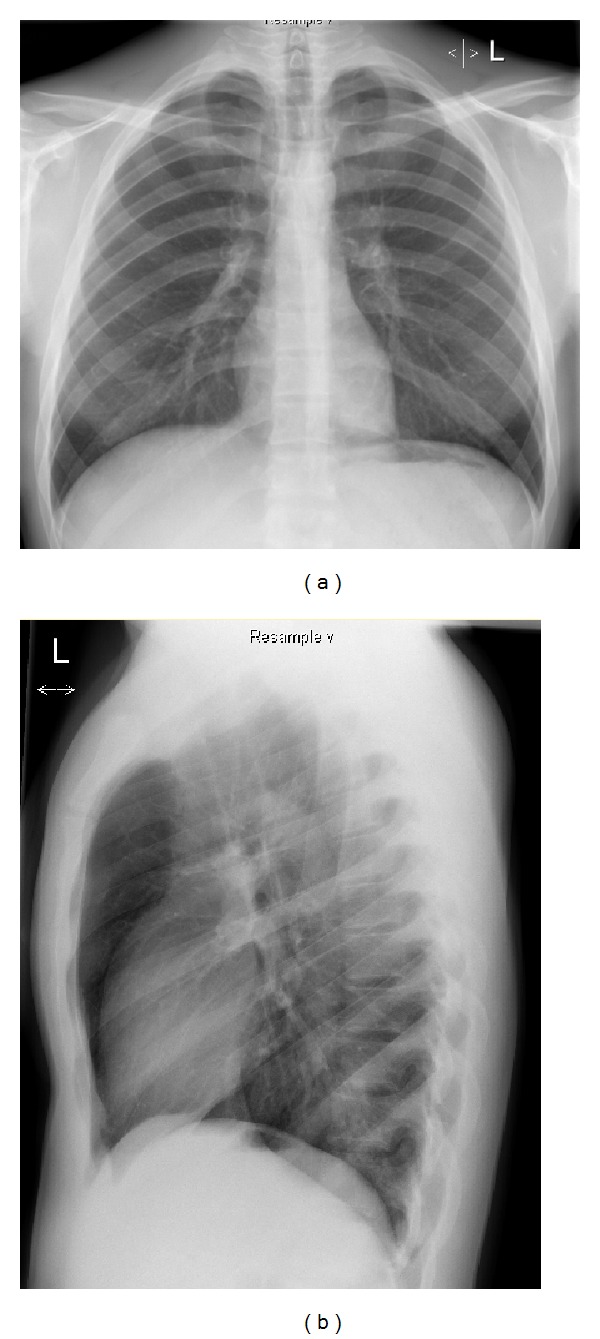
Chest X-ray showing complete resolution of findings compared to Figures 1(a) and 1(b).
